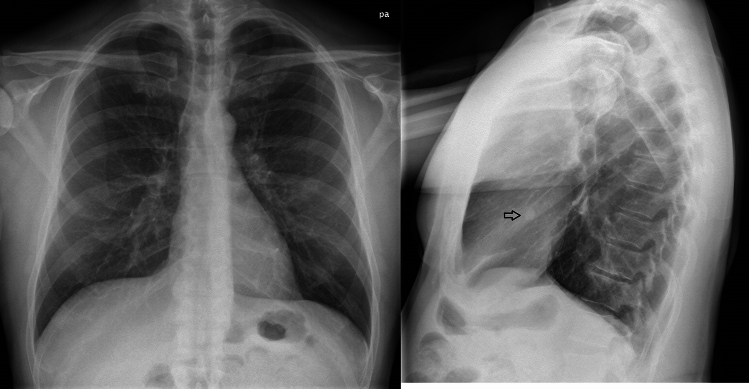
Chest X-ray (CXR) of a patient with 12 metastatic nodules visible in ULD-CT at 2 months. CXR shows the largest nodules (12 mm).
